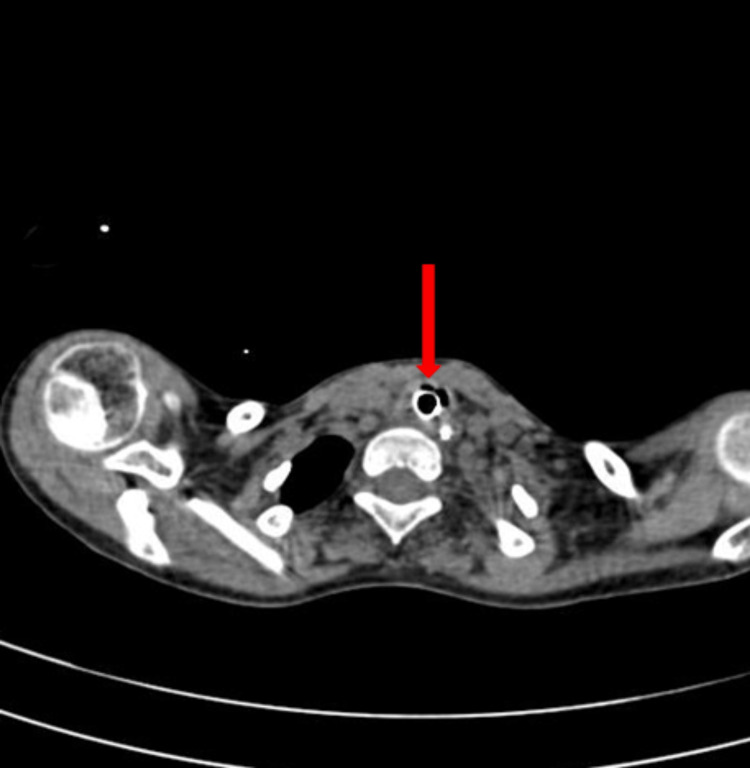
HRCT thorax showing tracheal deviation from the midline to the left side (indicated by the red arrow) HRCT: High-resolution computed tomography
